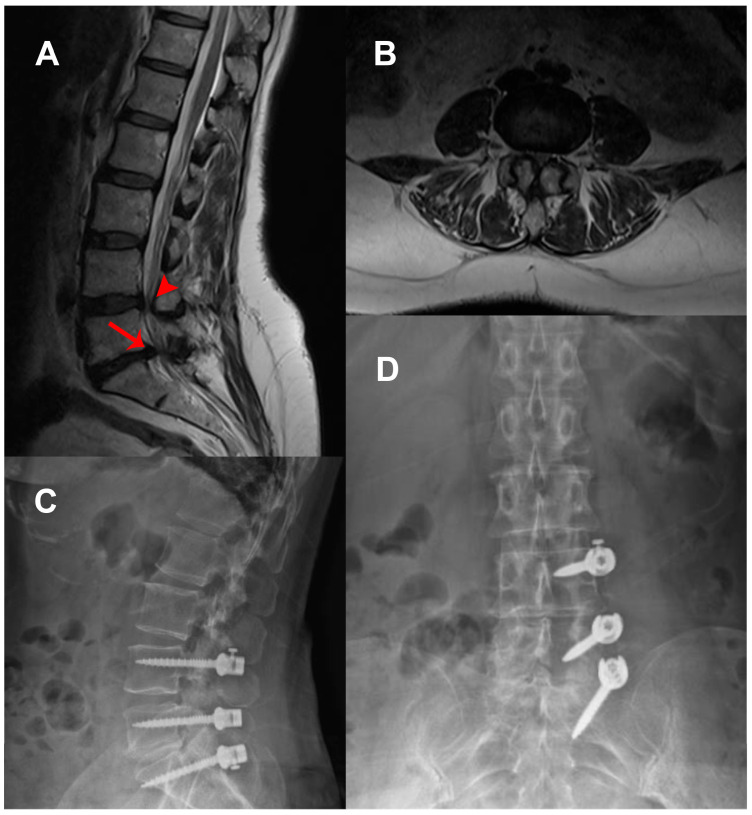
A 54-year-old female patient presented with new-onset lower back pain and bilateral leg pain, more pronounced on the left, accompanied by left leg hypoesthesia. (A) Sagittal and (B) axial T2-weighted MRI demonstrating spinal stenosis (arrowhead) at L4–L5 and disc herniation (arrow) at L5–S1. The patient underwent L4–S1 decompression and L5–S1 microdiscectomy, followed by left-sided L4–S1 stabilization using dynamic screws with a PEEK rod. Postoperative standing radiographs: (C) lateral and (D) anteroposterior views confirming stable dynamic fixation.
